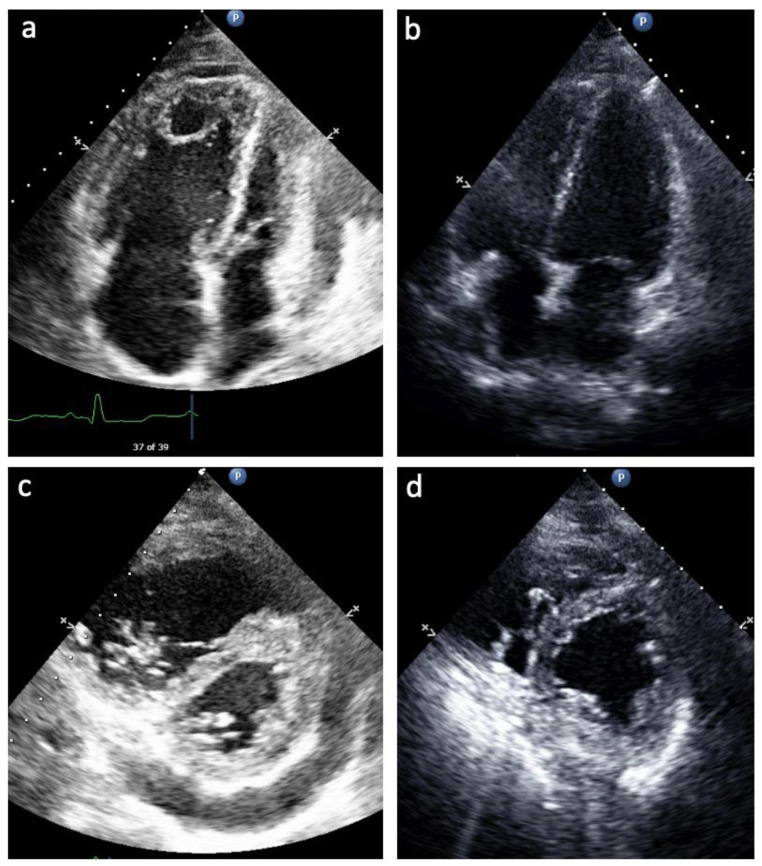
Echocardiogram from Patient 3 prior to (a,b) and after (c,d) PAH medication optimization. (a) Apical 4 Chamber view of enlarged RA and enlarged and hypertrophied RV with small and underfilled LV, LA; (c) Apical 4 Chamber view of normalized RA and RV size and function on PAH therapy; (b) Parasternal short axis view of severe systolic septal flattening, RV enlargement and hypertrophy, and pericardial effusion; (d) Parasternal short axis view of resolution of systolic septal flattening and pericardial effusion with smaller RV size.
